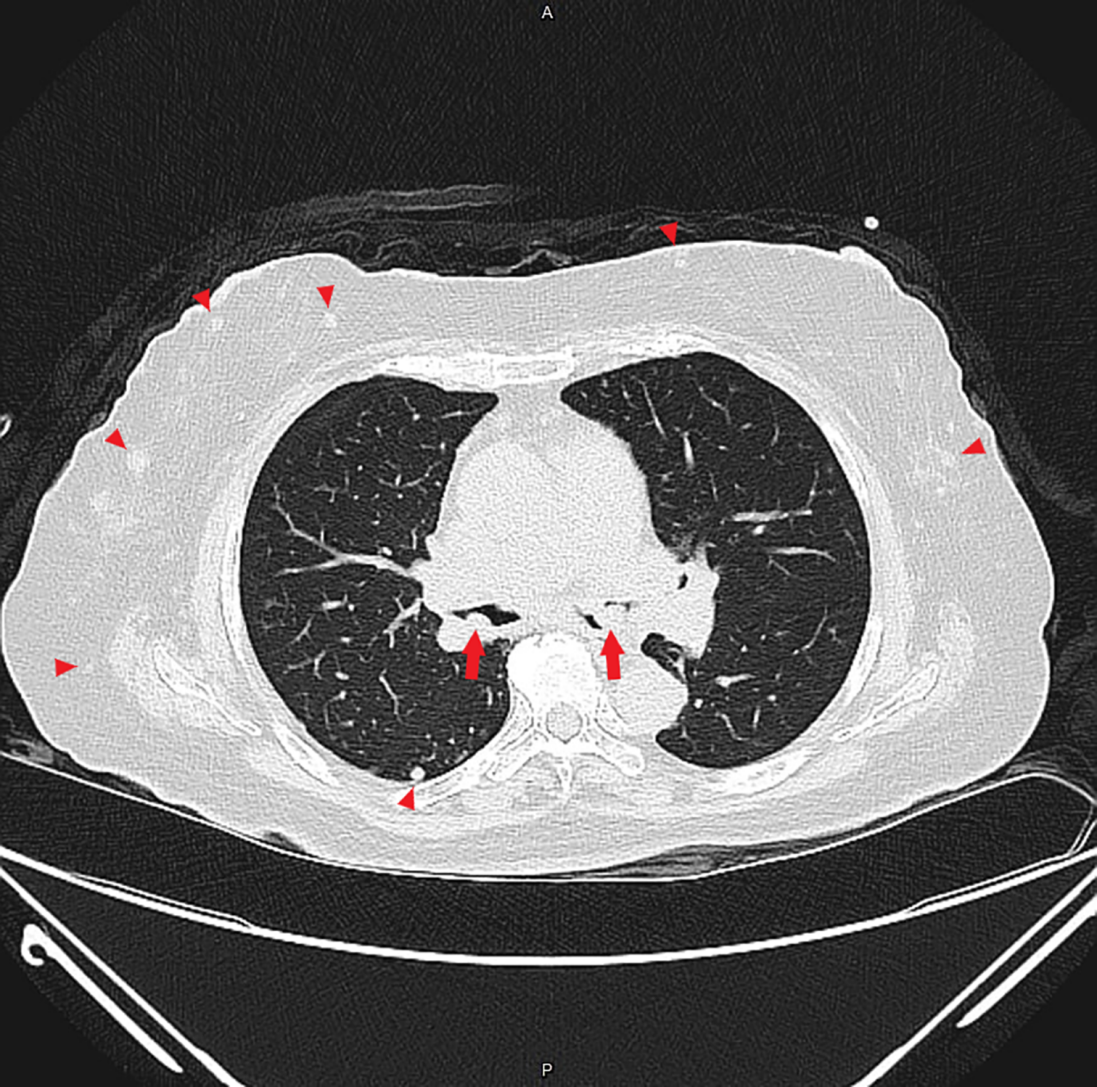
Non‐contrast chest CT showed multiple nodular lesions (arrowheads) in the subcutaneous tissue and lungs. Additionally, multiple endobronchial lesions (arrows) were also observed.
